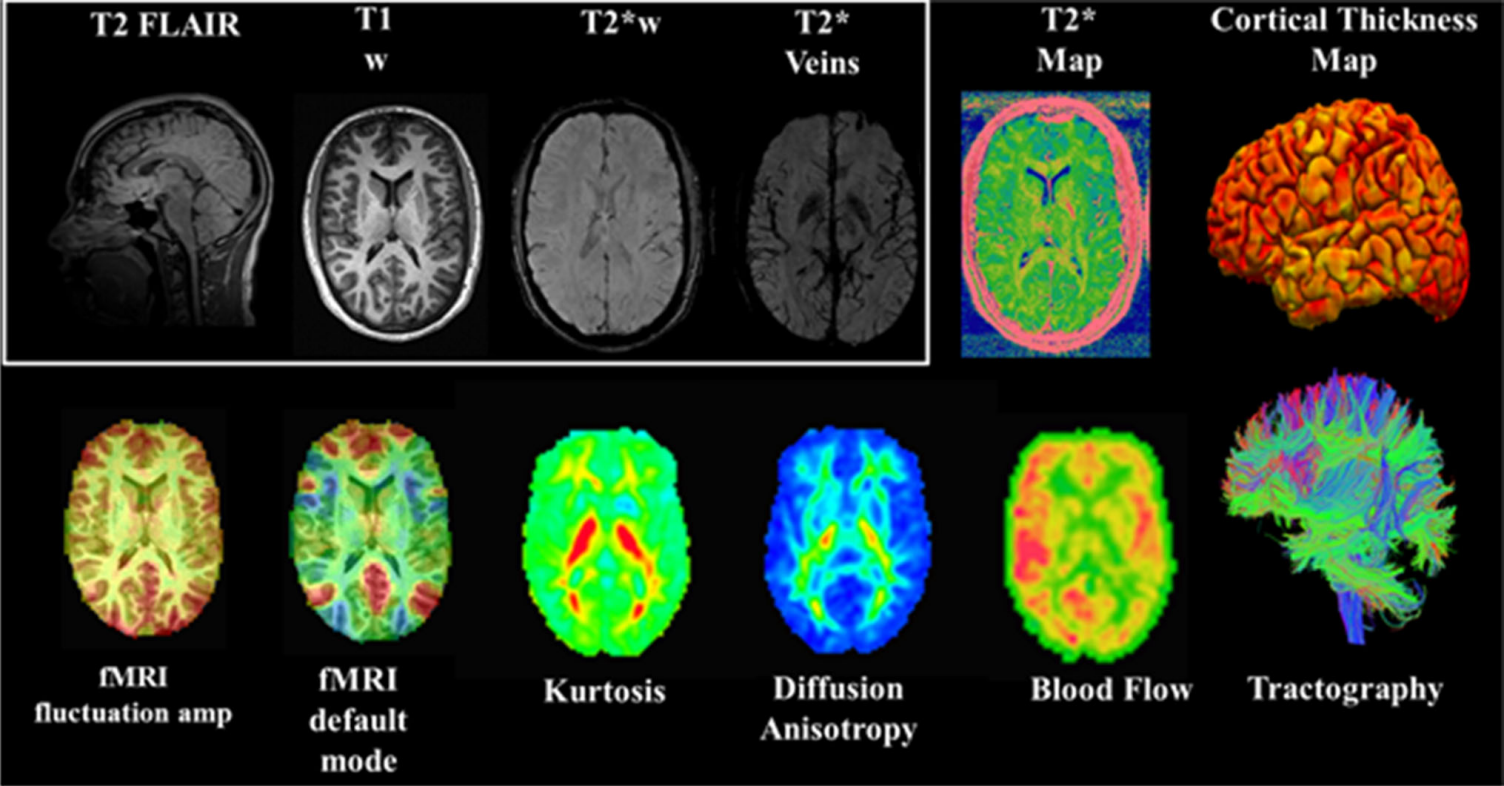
CARE ARC MRI protocol integrating multimodal imaging biomarkers of changes in brain structure and function. *CARE* Concussion Assessment, Research, and Education, *ARC* Advanced Research Core, *MRI* magnetic resonance imaging
